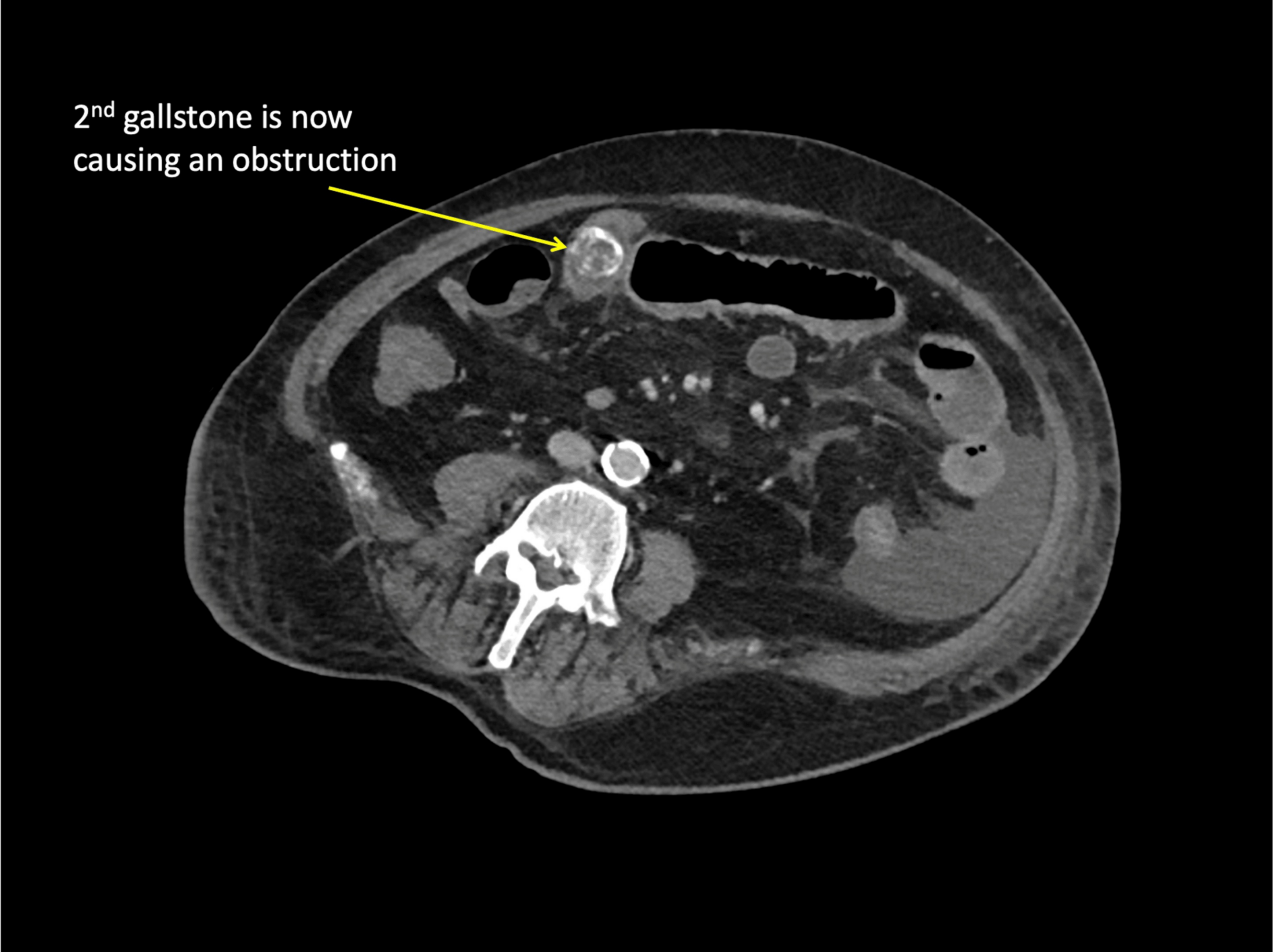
Axial CT showing recurrent gall stone ileus.
